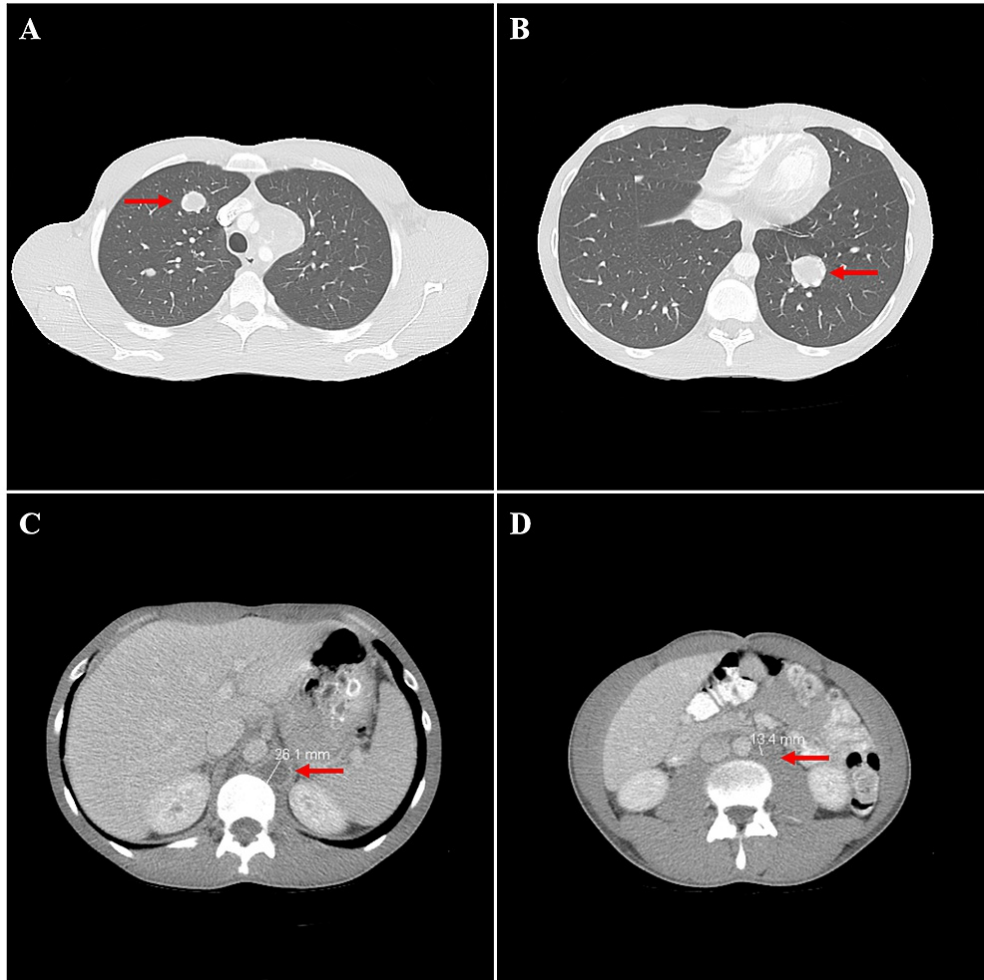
CT chest during patient’s hospital admission showed a left lower lobe lung mass measuring 2.8 cm (A) (red arrow) and a large heterogeneously enhancing mass in the anterior upper mediastinum consistent with metastatic disease (B) (red arrow). CT abdomen and pelvis showed left retrocrural adenopathy measuring up to 2.8 cm in the upper abdomen (C) (red arrow) and para-aortic lymphadenopathy with lymph nodes measuring up to 1.7 cm (D) (red arrow) CT: computed tomography
